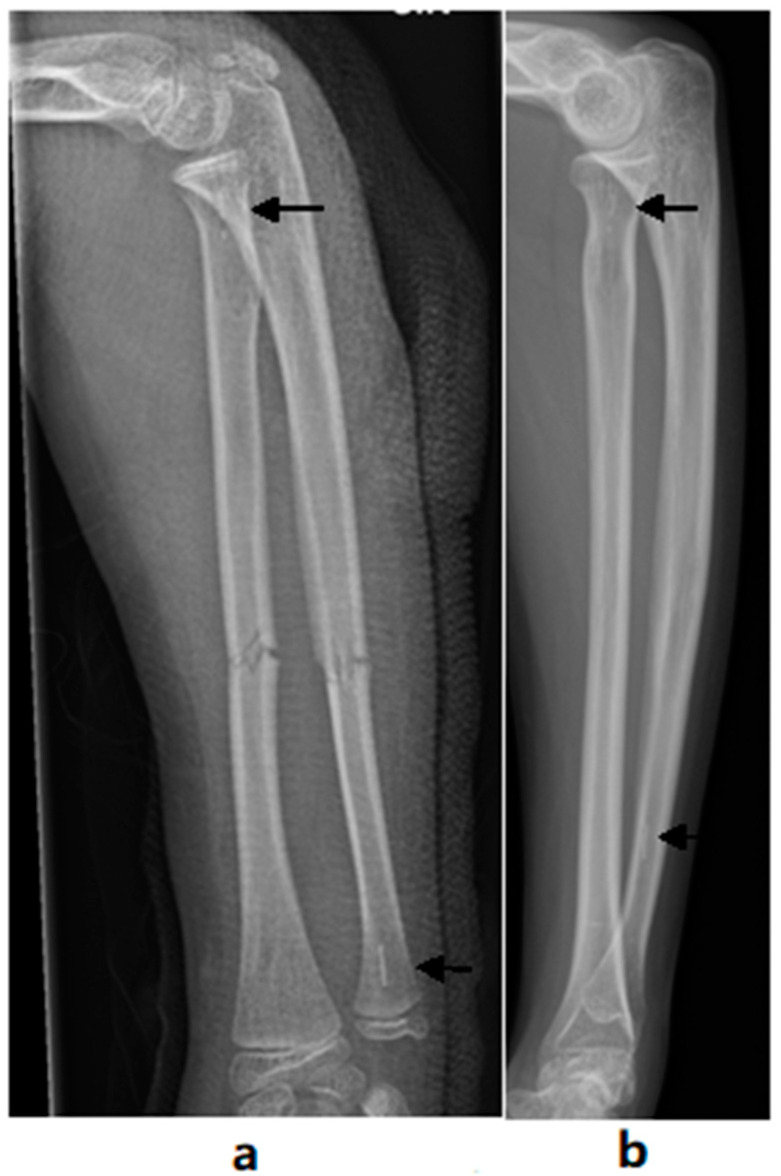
Forearm radiographs with radiolucent β-TCP-tips (black arrows) of the biodegradable nails immediately after operation (a) and 6.6 years after operation (b).
